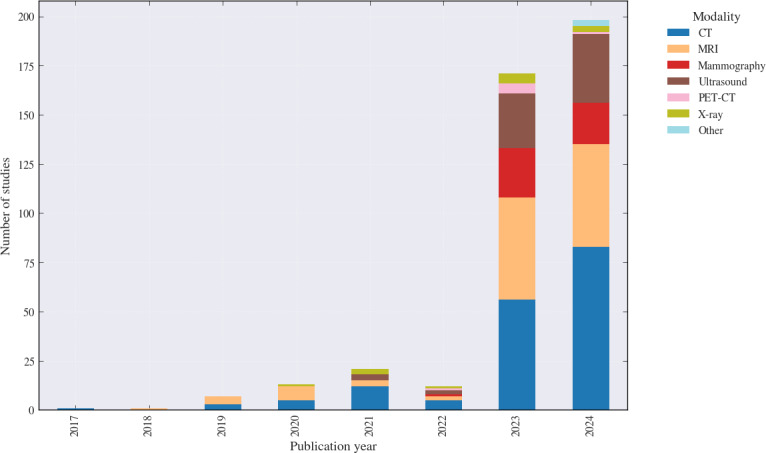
Distribution of imaging modalities used in explainable artificial intelligence studies for cancer imaging (2017‐2024; N=371). Stacked bars indicate the number of studies per publication year by modality. CT: computed tomography; MRI: magnetic resonance imaging; PET: positron emission tomography.
